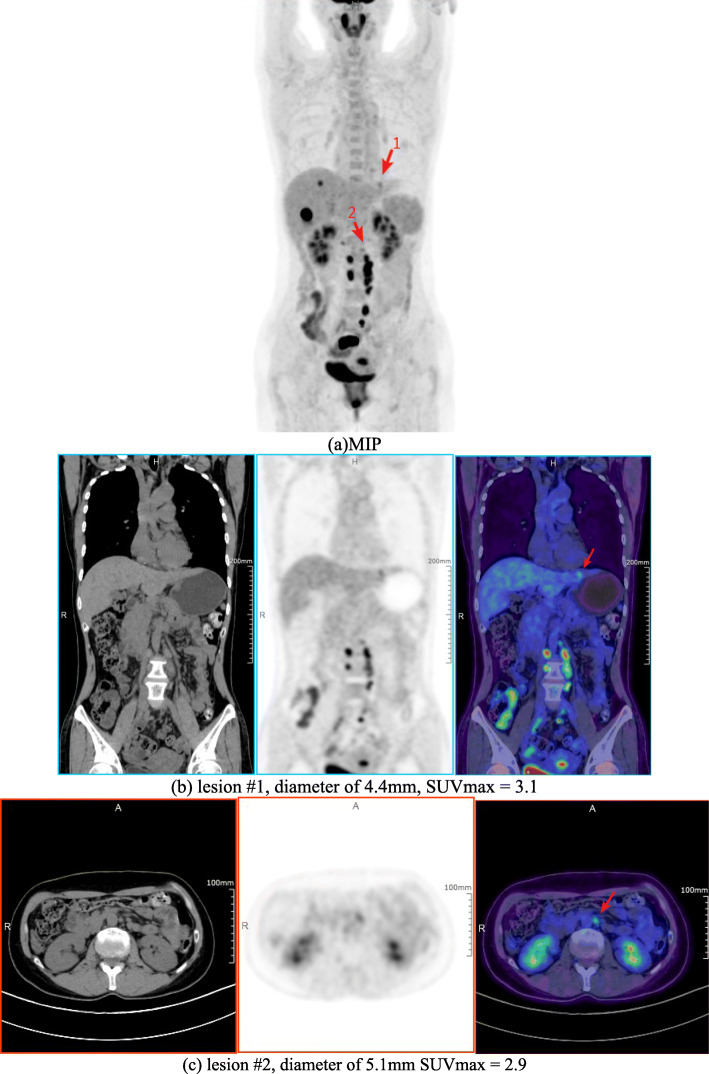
Second patient images. a MIP, two small lesions were pointed out by red arrows. b Lesion #1 images, from left to right, CT, PET, and PET/CT fusion image. c Lesion #2 images, from left to right, CT, PET, and PET/CT fusion image
